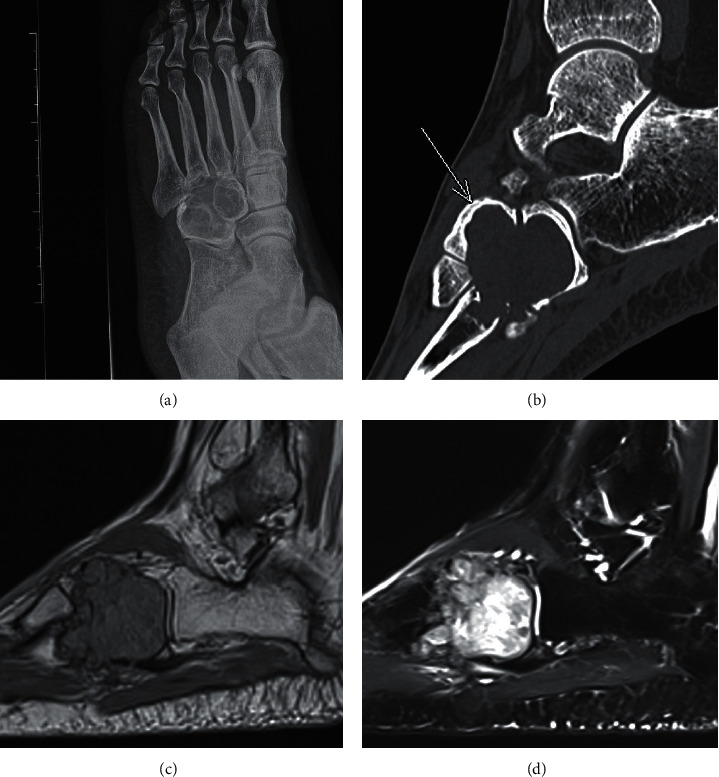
(a) Plain X-ray of left foot demonstrated an expansile lytic lesion in cuboid and lateral cuneiform. (b) CT scan highlighted its well-defined margin and cortical scalloping of lateral cuneiform (arrowed). (c) MRI showed PD-intermediate signal of the lobulated lesion with distal cortical perforation and (d) hyperintense signal on T2.
